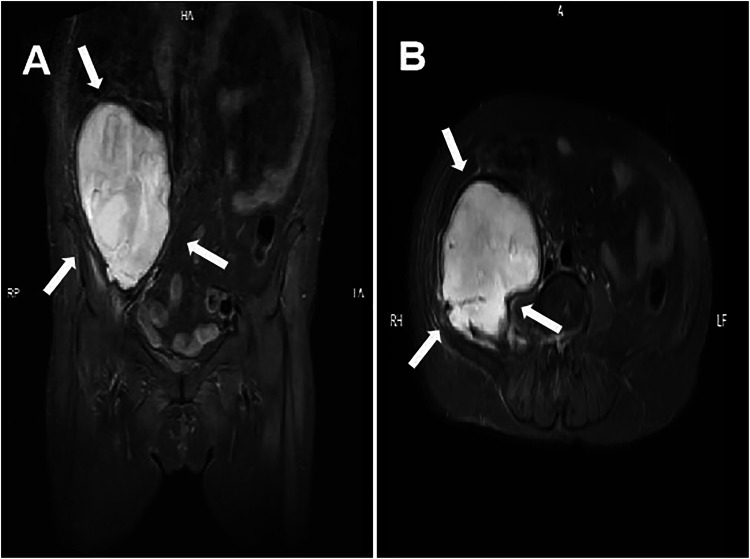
Four weeks after arterial embolization, MRI revealed a retroperitoneal hematoma with minimal resorption (arrows). (A) Coronal plane; (B) cross-section.
